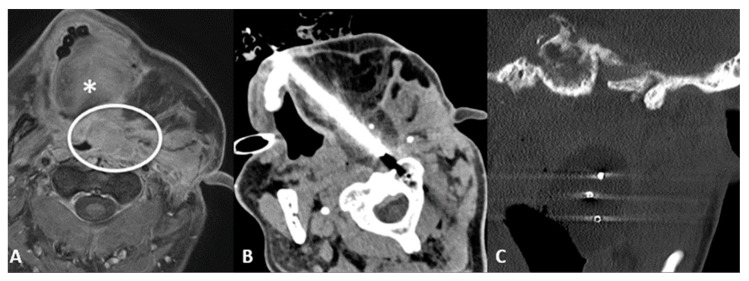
70-year-old male with airway impairment and left postauricular discharge. Previous nasopharyngeal carcinoma and radiation-induced squamous cell carcinoma of maxilla which underwent maxillectomy, mandibulectomy, parotidectomy, and myocutaneous flap reconstruction. (A) T1-weighted postcontrast axial MRI scan showing extensive tumor recurrence (white circle), posterior to the tongue (*), threatening the airways and invading the left external auditory canal. (B) Axial CT scan showing cryoablation needle placement through the myocutaneous flap. (C) Coronal-oblique multiplanar reconstruction CT scan showing the ice ball formed by the three cryoprobes.
